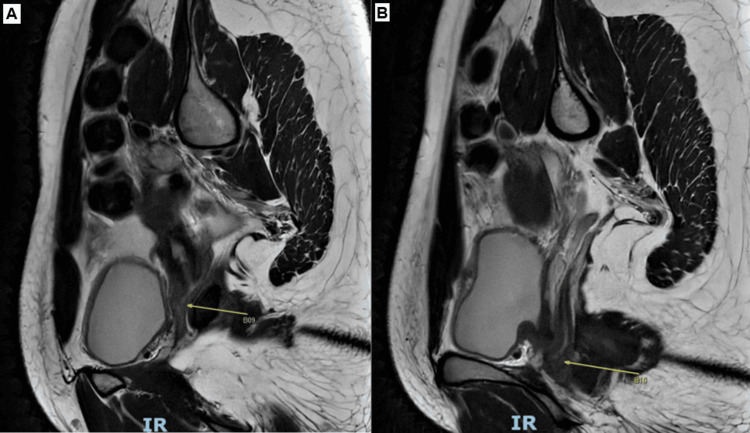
(A, B) MRI image showing the left uterine horn communicating with the left cervix and vagina MRI: magnetic resonance imaging
